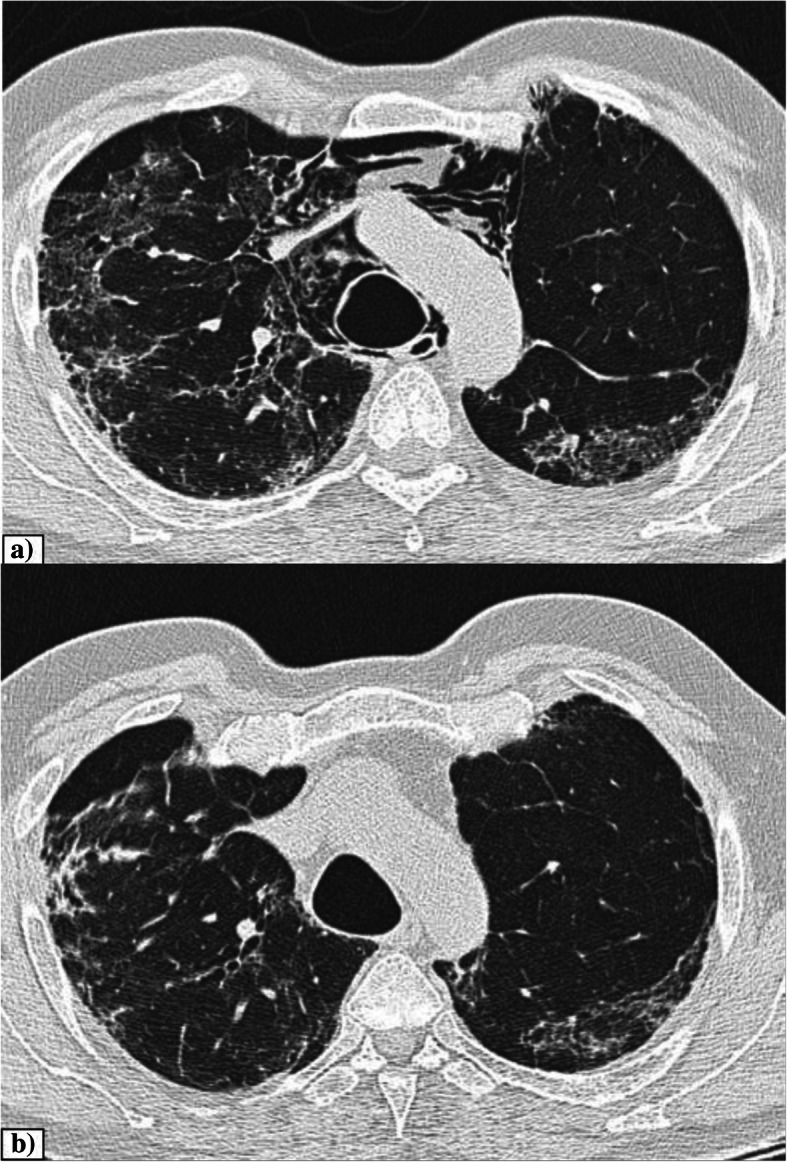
Spontaneous pneumomediastinum. a Axial CT thorax of a 58-year-old COVID pneumonia patient showing pneumomediastinum in the pre-vascular space and around trachea and esophagus. Visualized lung shows peripheral GGOs with interlobular septal thickening. b Complete resolution of pneumo-mediastinum after 10 days
